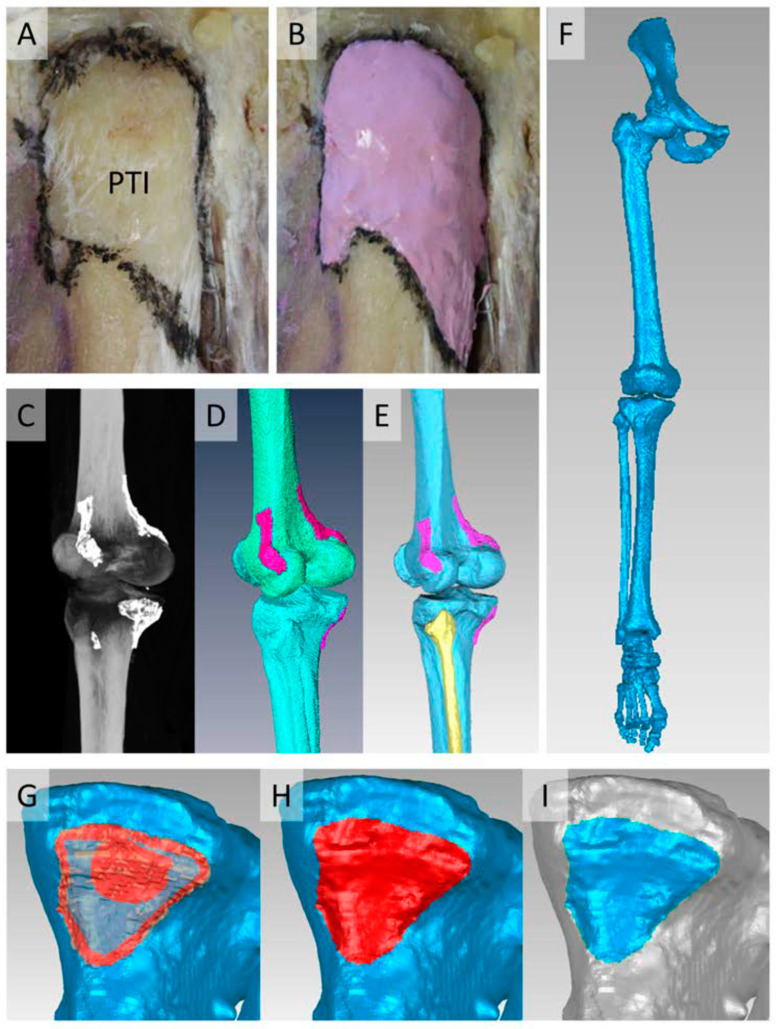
The process of reconstruction of bones and attachment markings is as follows: (A) Contour of the patellar tendon insertion (PTI) footprint at the anterior tibia after dissection by a surgical marker. (B) Painted footprint of the PTI with radiopaque paint. (C) Radiopaque markings of attachments during CT. (D) Rough surfaces after the segmentation process. (E) Smoothed and corrected bone surfaces with unedited paint surfaces. (F) Coherent model with all bones in position after fixation. (G–I) Process of footprint reconstruction. (G) Tracing of contact area through a transparent paint surface on the bone. (H) Fully traced contact area on a bone. (I) Generated footprint surface on the bone.
